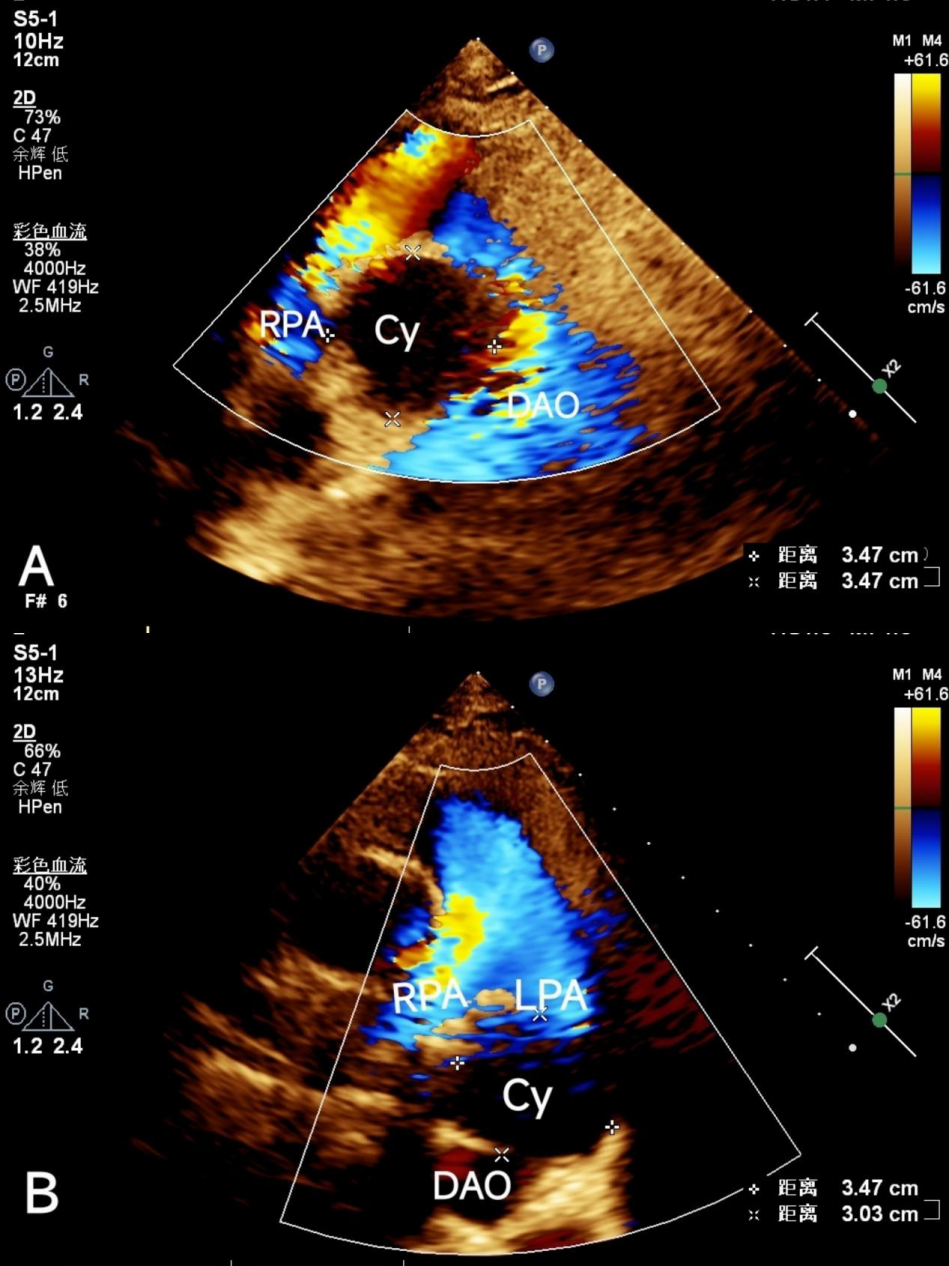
Echocardiographic imaging showing the precise localization of the cyst. (Cy: cyst; RPA: right pulmonary artery; LPA: left pulmonary artery; DAO: descending aorta).
